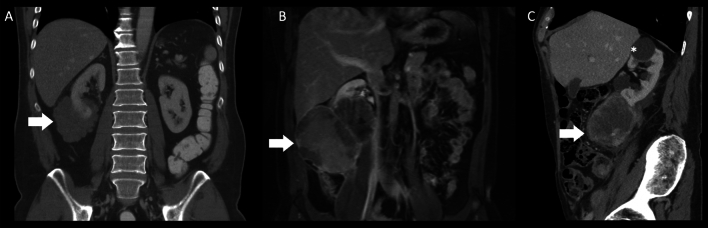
Representative radiological cases of primary renal angiosarcoma (A), primitive neuroectodermal tumor (PNET; B) and liposarcoma (C), each at the right lower renal pole. Subfigure A: coronal contrast-enhanced CT study demonstrating a lobulated, hypodense expophytic angiosarcoma (8.5 cm diameter; arrow). (B) coronal contrast-enhanced T1-weighted MR study showing an inhomogenously enhancing exophytic PNET (14.3 cm diameter; arrow). (C) sagittal contrast-enhanced CT study revealing a peripherally enhancing exophytic liposarcoma (8.7 cm diameter; arrow). A renal cyst is noted at the right upper renal pole (asterix).
